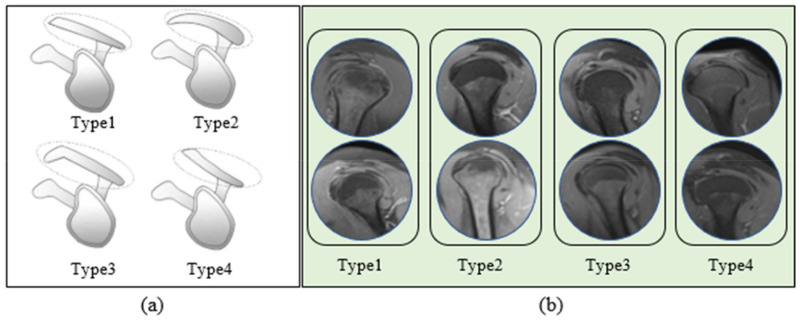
Graphical representation of acromion subtypes (a) and sample images of the shoulder MRI data set’s (b). Figure 1a presents a graphic representation of acromion subtypes . Moreover, Figure 1b presents (a) and sample images of the shoulder MRI data set’s Type 1, Type 2, Type 3, and Type 4 classes (b).
